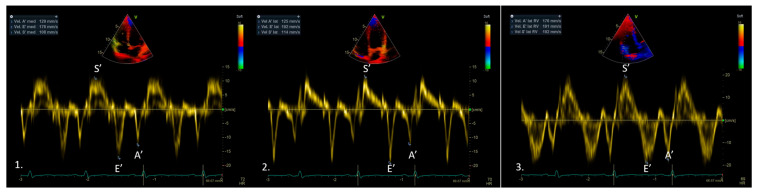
Tissue Doppler imaging (TDI) of a normal subject, obtained at the level of the inferior septum (1) and lateral wall (2) of the left ventricle, and the lateral wall of the right ventricle (3), showing normal systolic (S’) and diastolic (E’ and A’) velocities.
